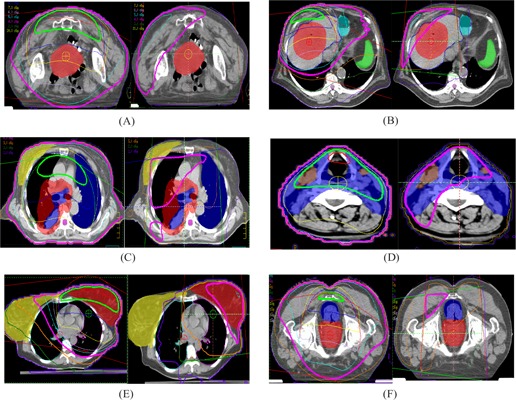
The two‐dimensional absolute dose distribution of transverse central slice is evaluated in various treatment sites—(A) pelvis, (B) abdomen, (C) lung, (D) head and neck, (E) breast, and (F) prostate—using megavoltage cone‐beam computed tomography images at 9 MUs (left panels) and orthogonal portal images at 4 MUs (right panels).
